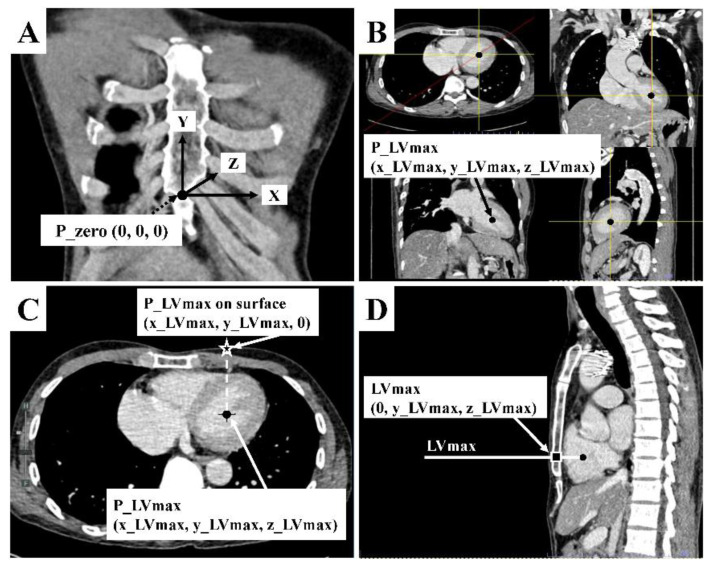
Chest computed tomography measurement. (A) The zero point (P_zero: [0, 0, 0]) was defined as the midpoint of the xiphisternal junction. It is the palpable lower end of the sternum where all sternal body, xiphoid process, and costal margins meet. From P_zero, the leftward, upward, and into the thorax directions of the patient were designated as positive on x, y, and z axes, respectively, which formed right angles to one another. (B) The P_LVmax (x_LVmax, y_LVmax, z_LVmax) was the midpoint of maximal left ventricular diameter in coronal, sagittal, and axial view. Its 3D coordinate was identified by using the picture archiving and communicating system’s function. (C) Assuming that P_LVmax was located on the anterior chest surface (Z = 0) just vertically above that midpoint, defined as P_LVmax on the surface (x_LVmax, y_LVmax, 0). (D) The sagittal view of CCT was used to locate the maximal left ventricular diameter on the sternum, defined as LVmax (0, y_LVmax, z_LVmax).
